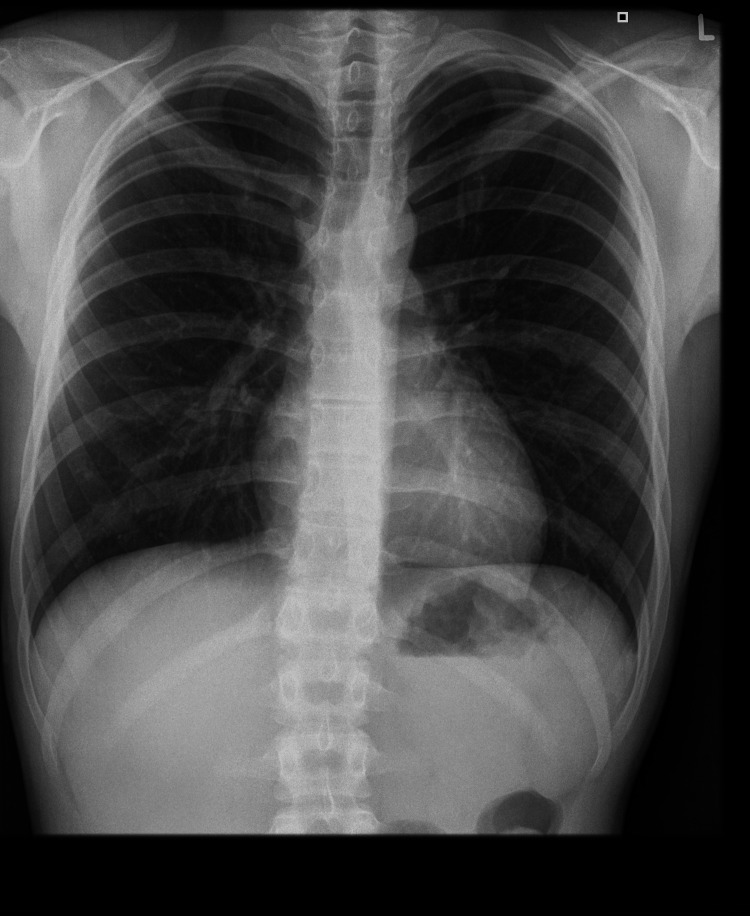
Normal chest posteroanterior (PA) X-ray
